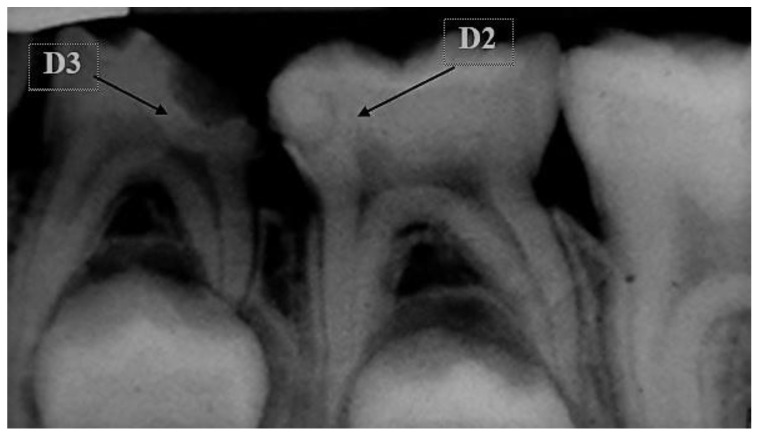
Preoperative periapical radiograph showing teeth 74 and 75. According to the American Dental Association Caries Classification System, tooth 74 showed disto-coronal radiolucency extending beyond the D3 level, i.e., into the pulp space and was treated with FSP. Tooth 75 shows mesio-coronal radiolucency extending beyond level D2, i.e., near the pulp horn and treated with IPT.
